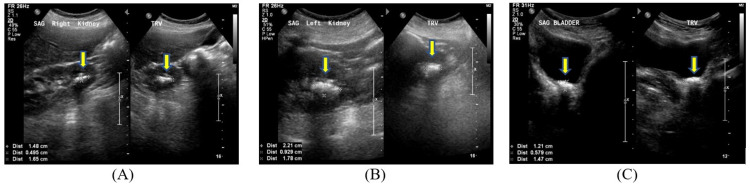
Transabdominal ultrasound of kidneys and urinary bladder Renal stones are present in the right kidney (A), left kidney (B), and left ureterovesical junction (C) (arrows)
